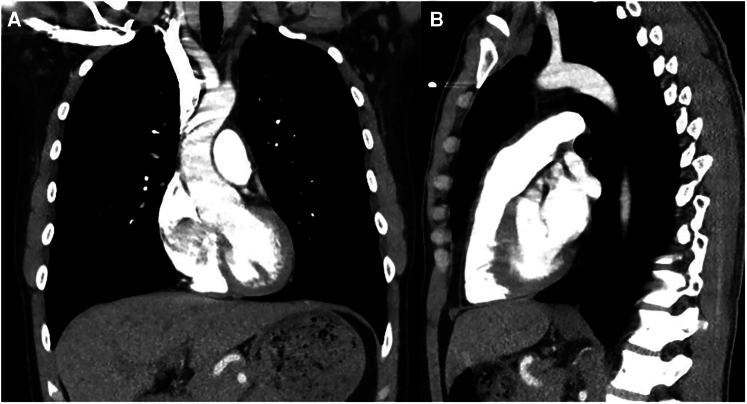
(*A*) Computed tomography chest coronal view demonstrating resolution of anterior mass. (*B*) Computed tomography chest sagittal view demonstrating resolution of anterior mass.
